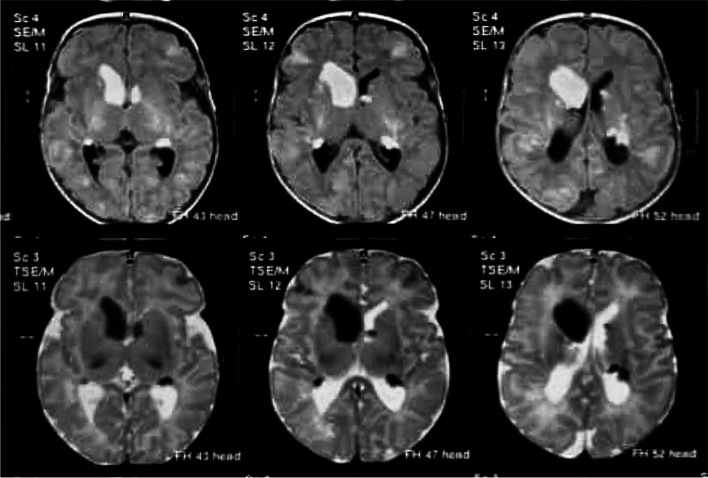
Axial T1-weighted and T2-weighted magnetic resonance images (MRI) showing high signal subepyndemal nodules at the frontal horn of the right lateral ventricle and in the occipital horns of both lateral ventricles
